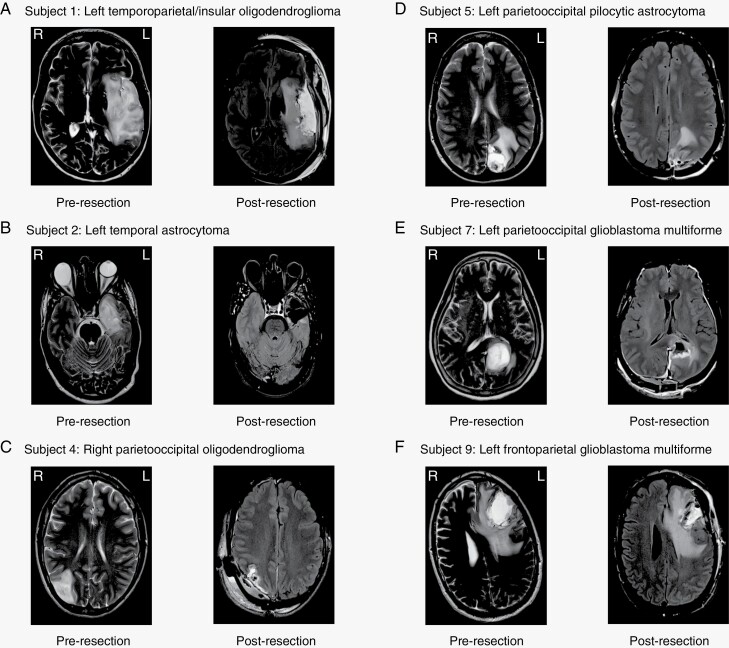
Pre- and post-resection neuroimaging. (A-J) Axial views of T2-weighted magnetic resonance imaging (MRI) (pre-) and T2-FLAIR (post) sequences for each study subject with delayed post-resection MEG recordings. Images were acquired before surgery and <48 hours following tumor resection. Note that the same imaging sequences are displayed for consistency. In some cases of high-grade tumors, edema, and post-operative mass effect may be more apparent.
